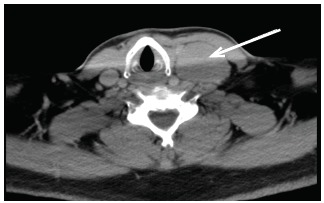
Computed tomography, axial image, demonstrating left level 2–5b neck adenopathy.
